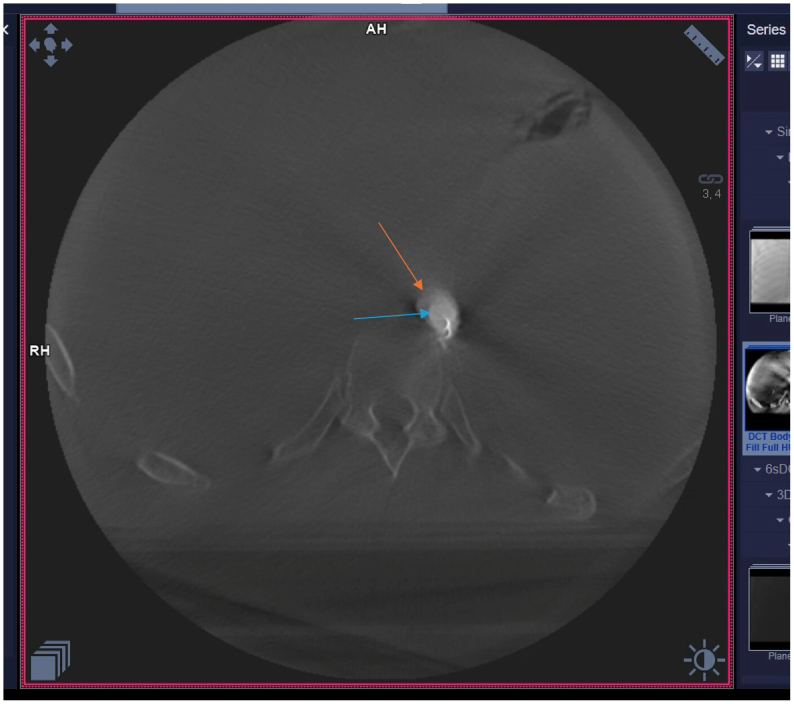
Intraoperative cone-beam computed tomography (CBCT) image portraying the creation of true and false lumens within the aorta. The *orange arrow* shows the false lumen of the aortic wall, and the *blue arrow* shows the true lumen made by the Dacron graft (DG) within the aorta.
